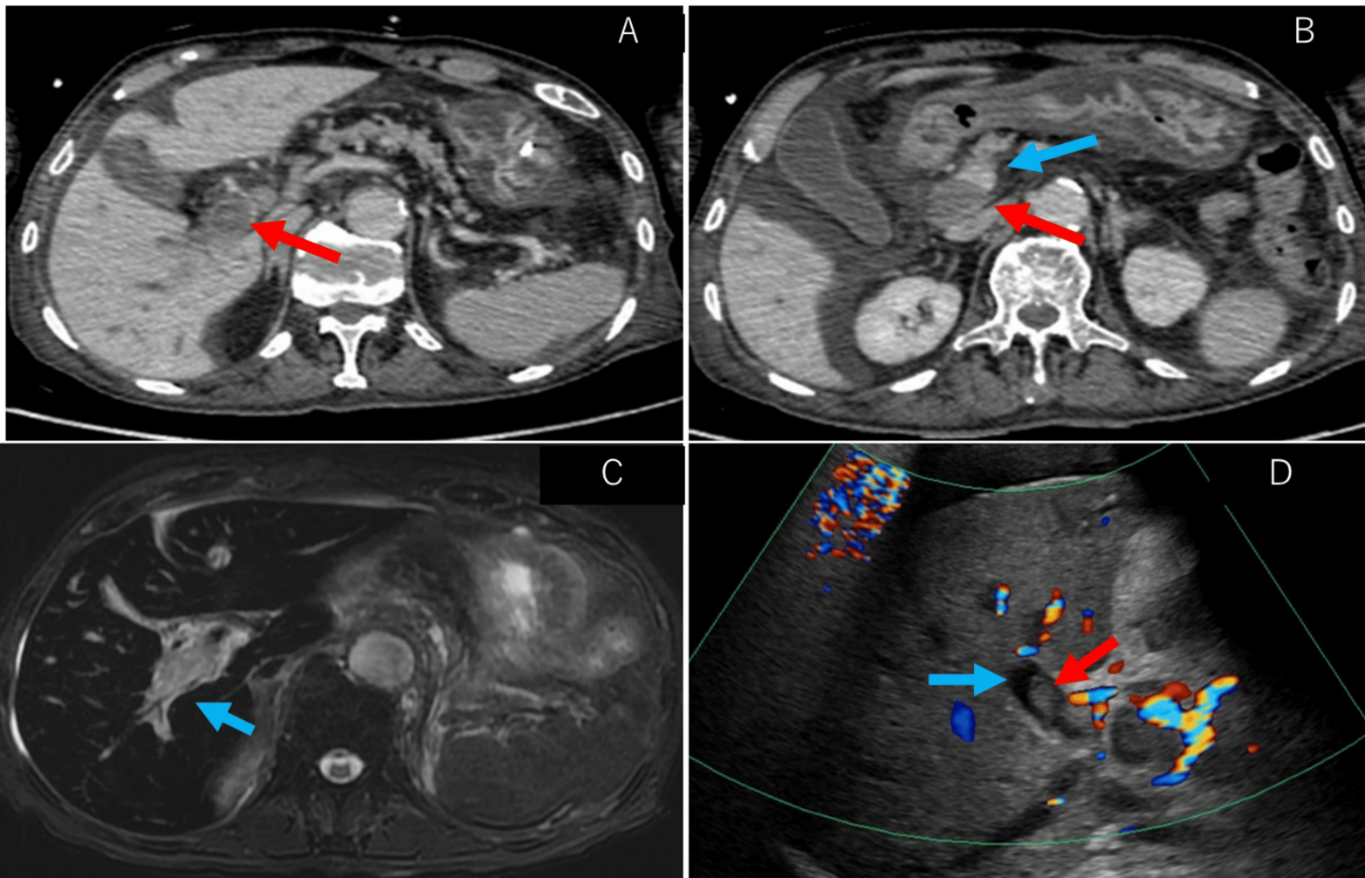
Abdominal computed tomography, magnetic resonance imaging, and ultrasonography (A and B) Contrast-enhanced computed tomography of the abdomen showed a portal vein thrombosis (indicated by the red arrow) in the main portal vein (indicated by the blue arrow). (C) The fat-suppressed T2-weighted abdominal magnetic resonance imaging scan showed a high-signal area along the portal vein, indicating the spread of inflammation. (D) Abdominal ultrasonography showed a thrombus (indicated by the red arrow) in the portal vein (indicated by the blue arrow). The flow within the portal vein had disappeared
